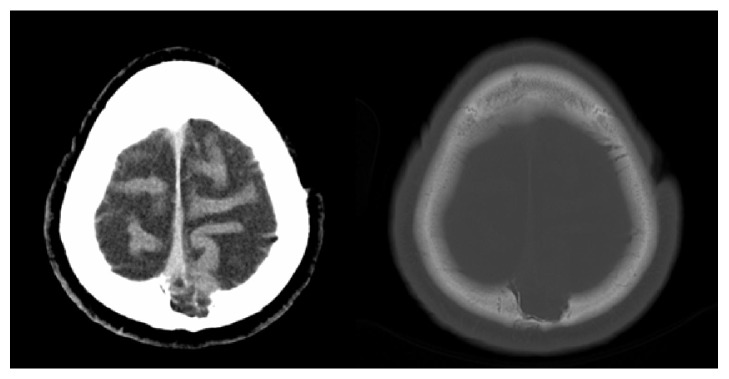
Head CT scan images show an incomplete bone defect in the left parietal bone, with heterogeneous density, including calcified areas.
